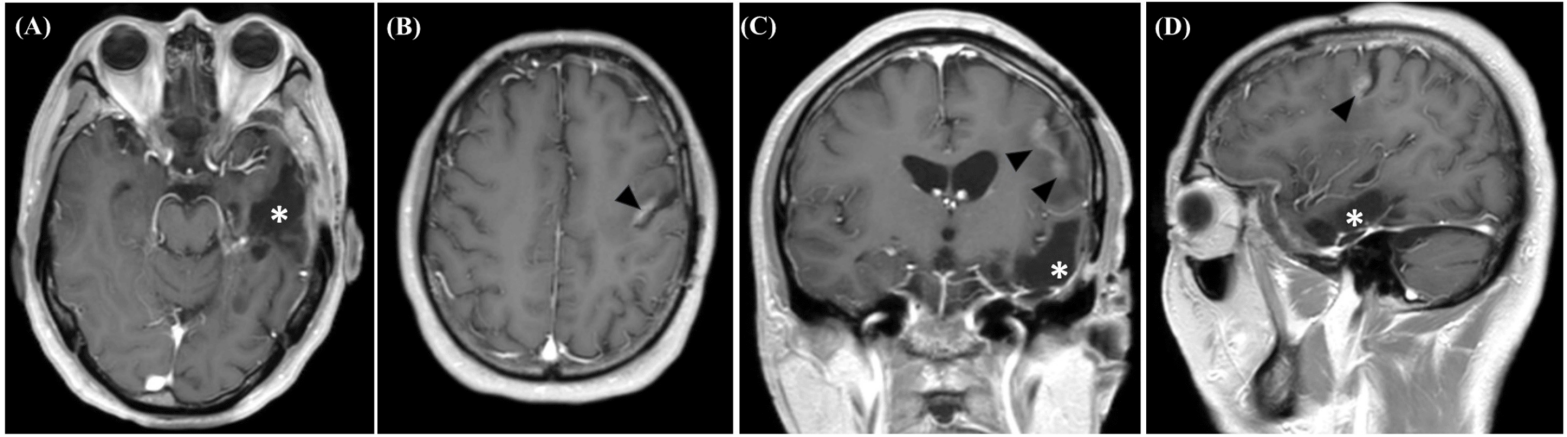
Follow-up MRI findings Brain MRI one year after surgery demonstrated no signs of tumor recurrence but changes following remote hemorrhagic infarction (arrowheads) on post-contrast T1WIs of axial (A and B), coronal (C), and sagittal (D) sequences. The asterisks indicate the surgical cavity. MRI: magnetic resonance imaging; WI: weighted image
